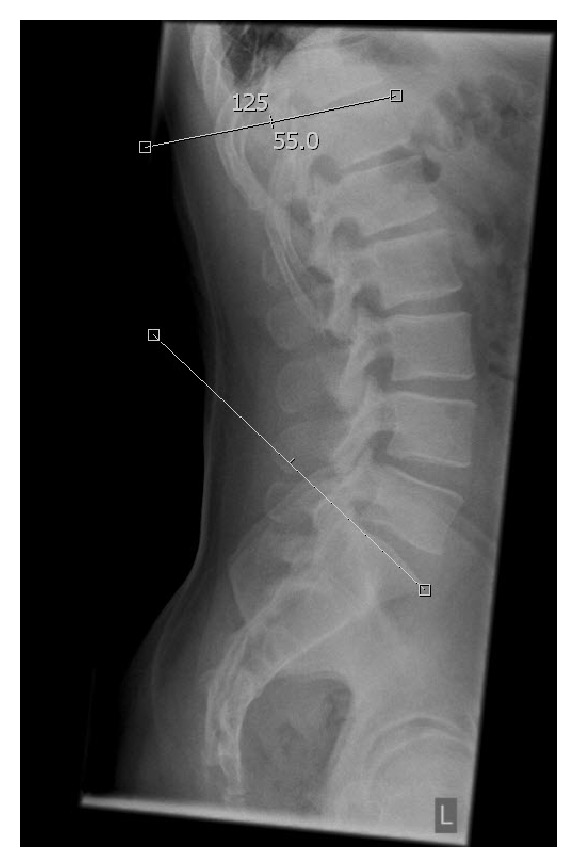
Functional Cobb angle measurement in conventional radiograph.
